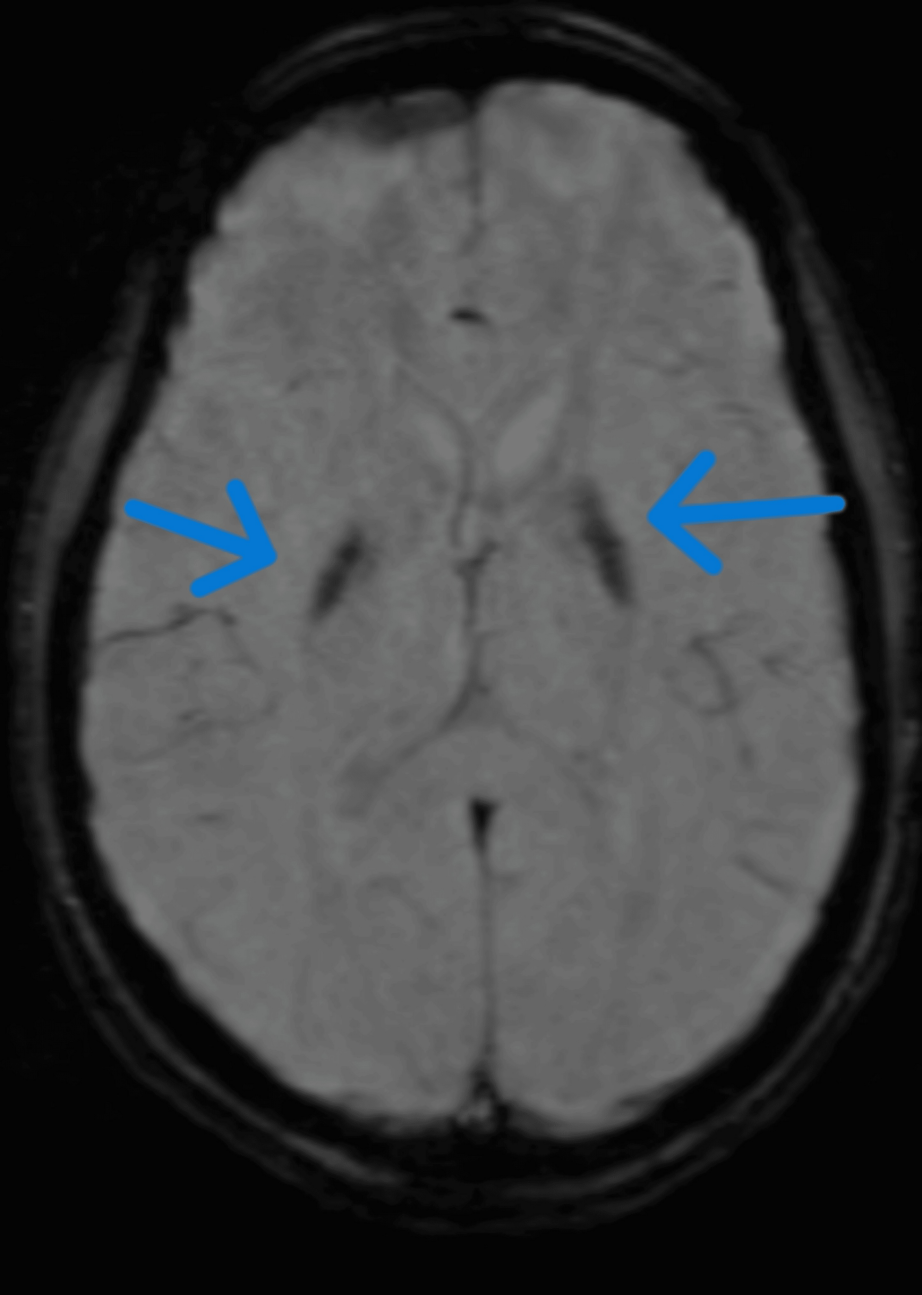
MRI brain susceptibility-weighted axial image showing blooming in bilateral basal ganglia. Blue arrow pointing toward bilateral basal ganglia.
